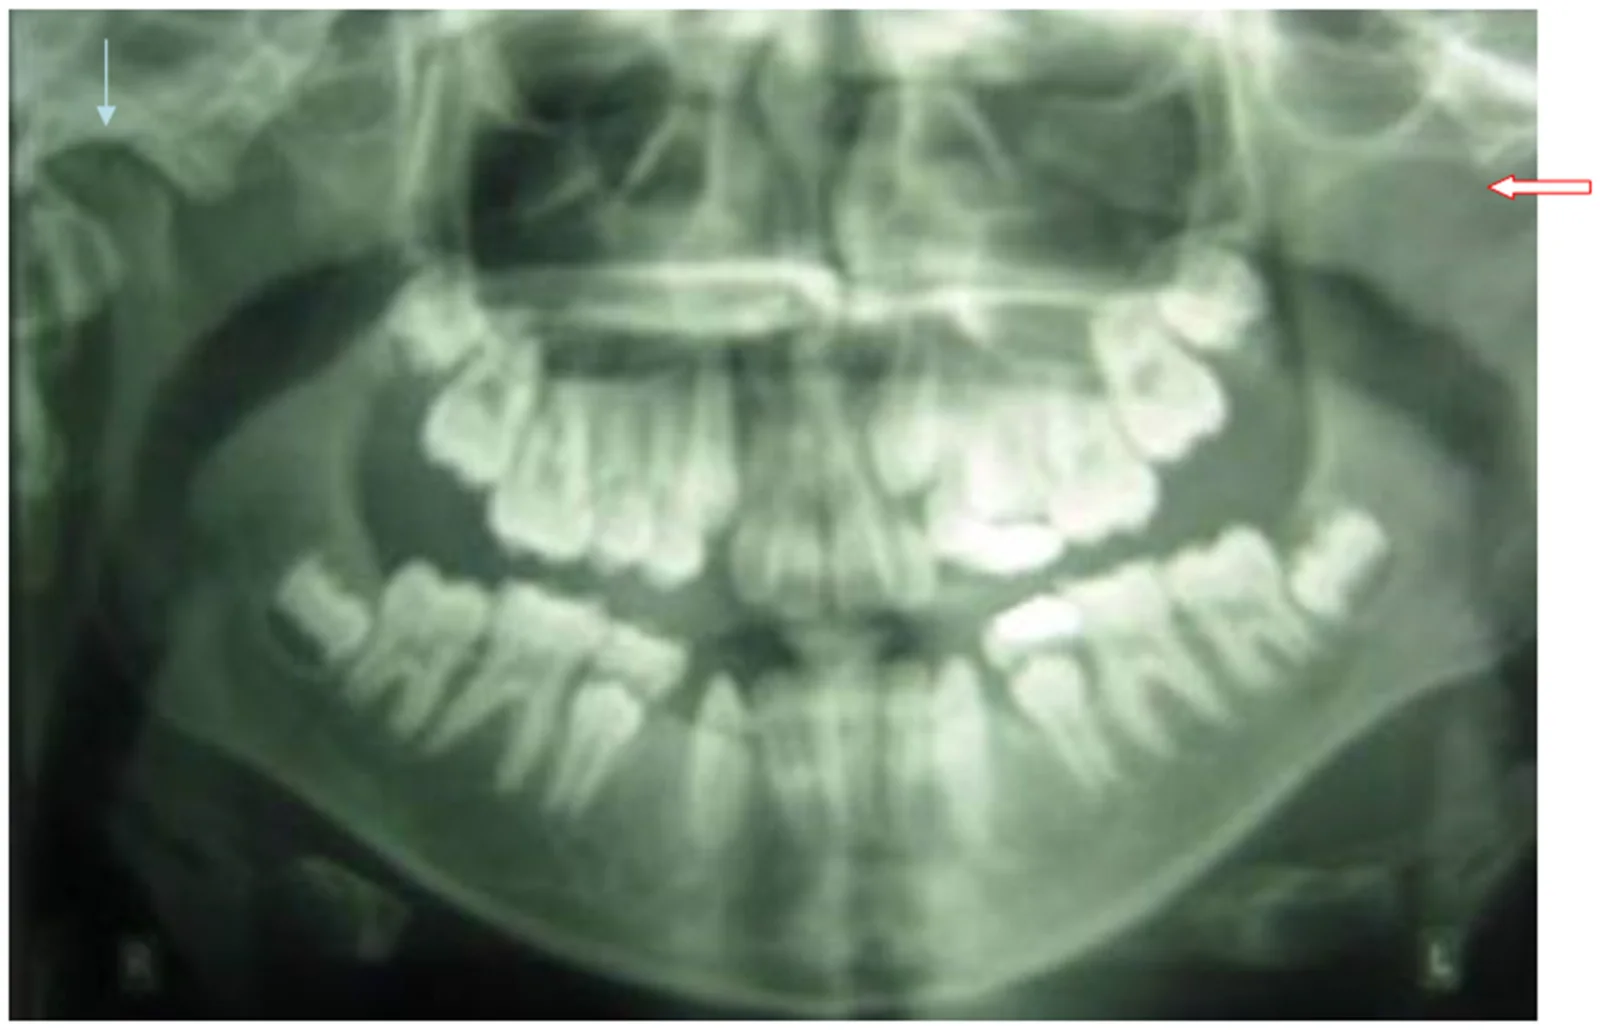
Panoramic radiograph of a female patient diagnosed with Juvenile Idiopathic Arthritis at the age of 12 years. In the right temporomandibular joint, the mandibular condyle (blue arrow) exhibits indistinct margins, irregular morphology, and a flattened, erosive articular surface, indicative of active bone degeneration. The left mandibular condyle (red arrow) is not adequately visualized, representing a limitation of the panoramic radiography that restricts detailed assessment of TMJ structural changes and may be attributable to a technical positioning error during image acquisition, precluding reliable evaluation of the left joint.
